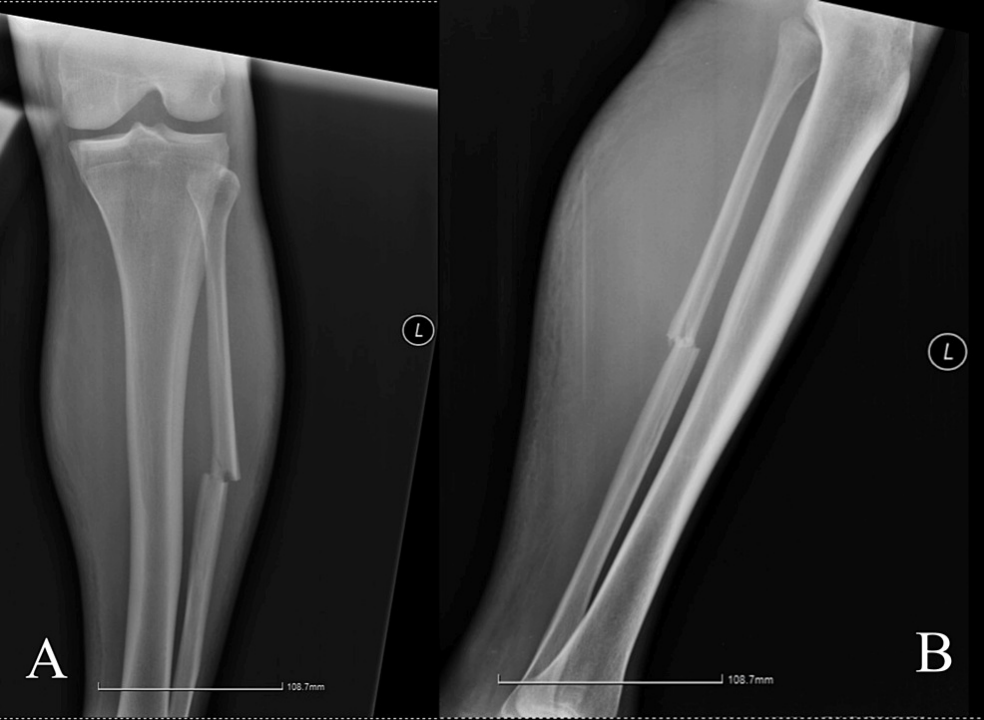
Left tibia radiograph showing anteroposterior (A) and lateral (B) views of the isolated fibular shaft fracture.
